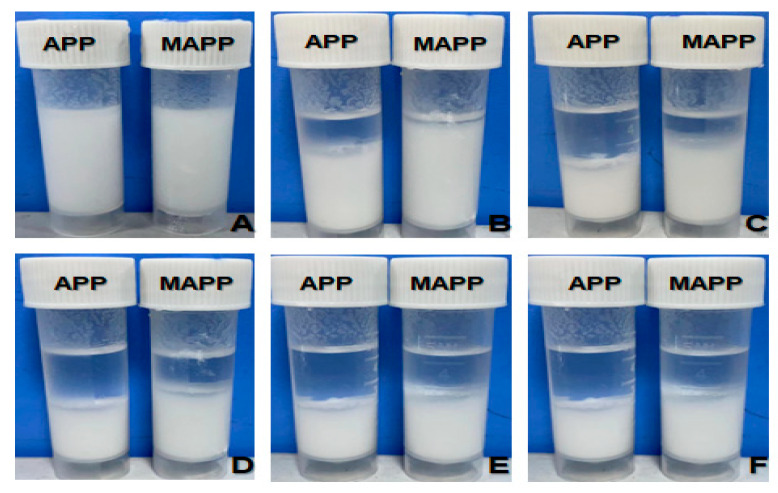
Settlement behavior of APP/MAPP powder in liquid paraffin at different times after ultrasound ((A): 0 h, (B): 0.5 h, (C): 3 h, (D): 12 h, (E): 48 h, (F): 72 h).
